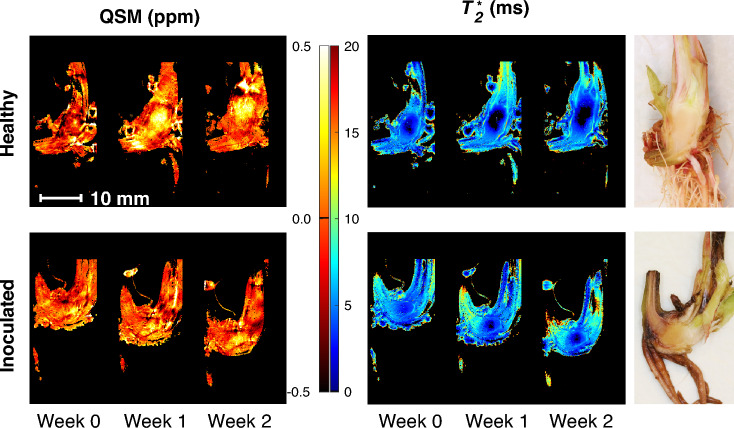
Example of QSM and $$T_{2}^{*}$$ relaxation time maps. Quantitative $$T_{2}^{*}$$ map and quantitative susceptibility map (QSM) of healthy and inoculated strawberry crowns during the two-week development period after inoculation. Mean of 10 consecutive slices from $$T_{2}^{*}$$ relaxation time maps and quantitative susceptibility maps (QSM) are presented. Averaging effectively provides the same slice thickness as obtained with $$T_{1}$$ fast spin echo multi slice—inversion recovery (FSEMS-IR) and $$T_{2}$$ multi echo multi slice (MEMS) data sets. Differences in the visibility of the stolon and root structures between time points are due to slightly different MR image slice positions. For comparison, similar positions with respect to the relaxation time maps in Fig. 3 were selected for these maps. The representative examples here are #4 from healthy and #1 from inoculated plantlets presented in Supplementary Fig. S1 online. Relaxation data from surrounding water have been omitted (black). For Fig. 4a, the color gradient represents magnetic susceptibilities from negative diamagnetic susceptibility (dark red) to positive paramagnetic susceptibility (light yellow), with zero susceptibilities in-between (orange). For Fig. 4b, the color gradient represents the relaxation times for each region, from relatively low relaxation time values (blue) to relatively high relaxation time values (red), and relaxation time values in-between (cyan, green, yellow and orange).
